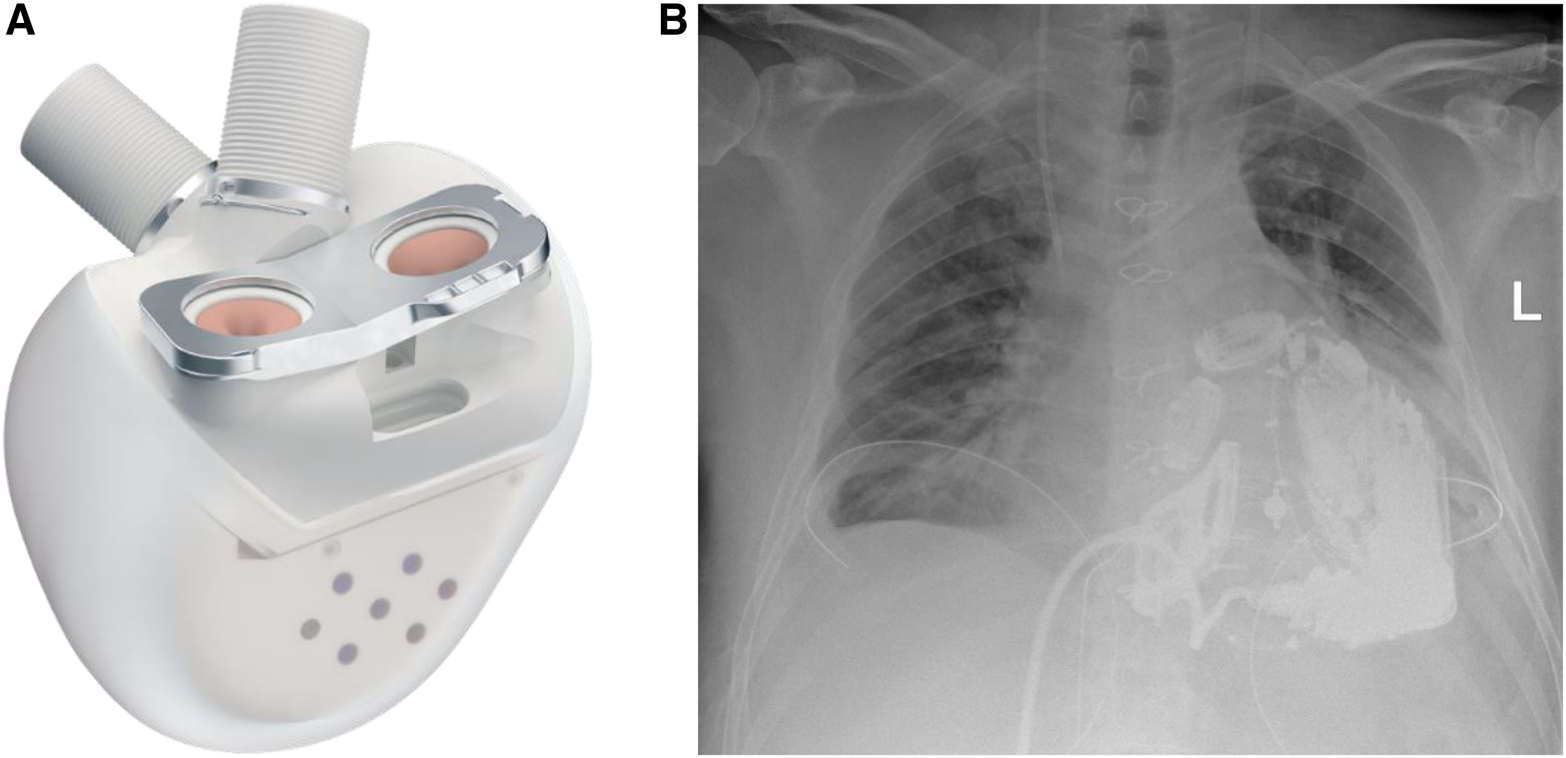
(A) The Aeson TAH device, courtesy of CARMAT (SA, Vélizy, France). (B) Chest x-ray of the patient after Aeson TAH implantation.
